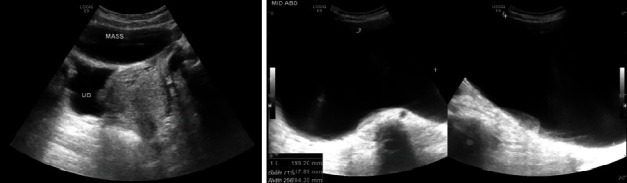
Abdominal and pelvic ultrasonography showed a huge cystic lesion with clear fluid contents.
